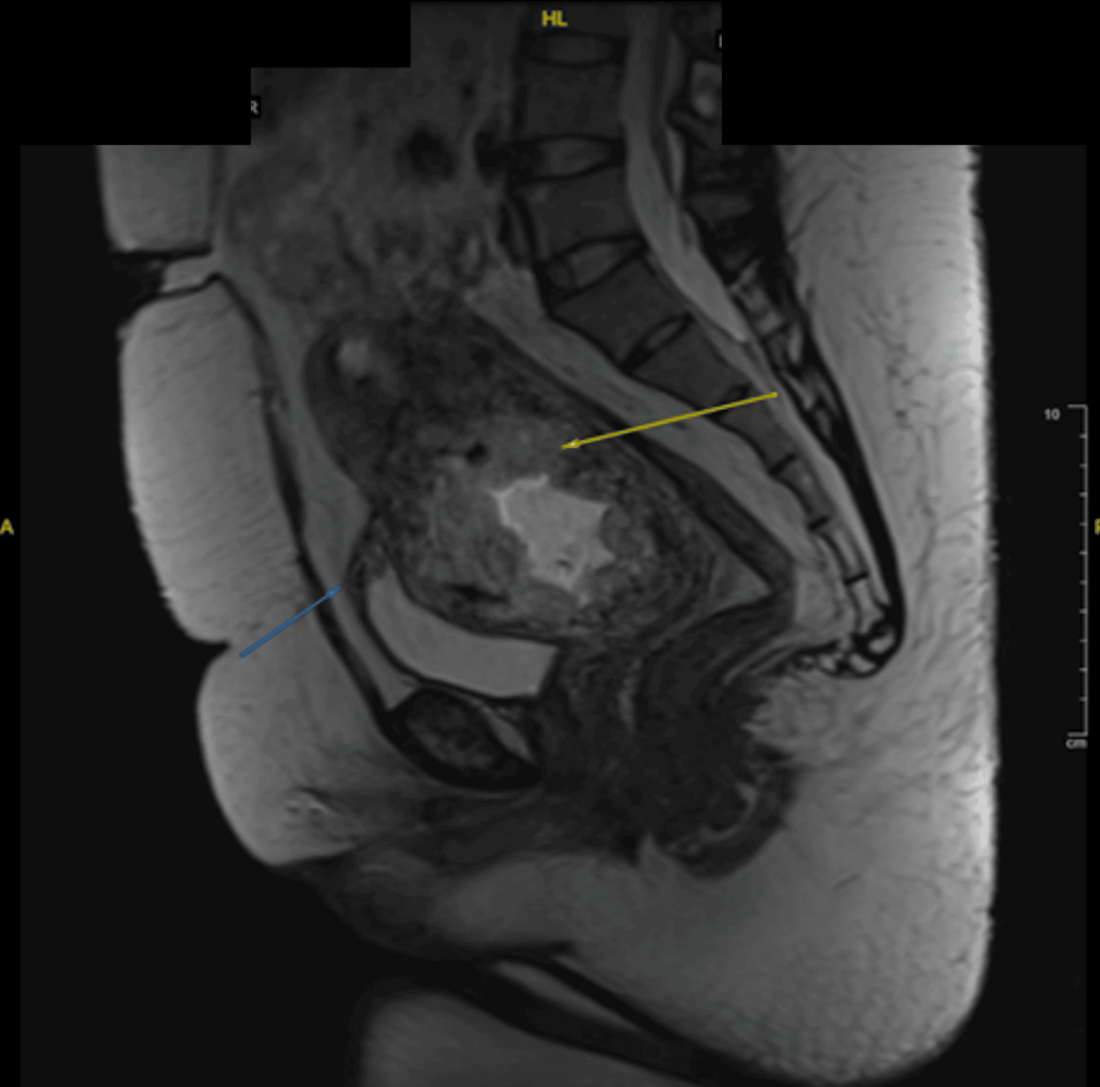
MRI upon diagnosis showing a likely molar pregnancy with serosal breach through the cesarean scar (yellow arrow) and urinary bladder invasion (blue arrow)
